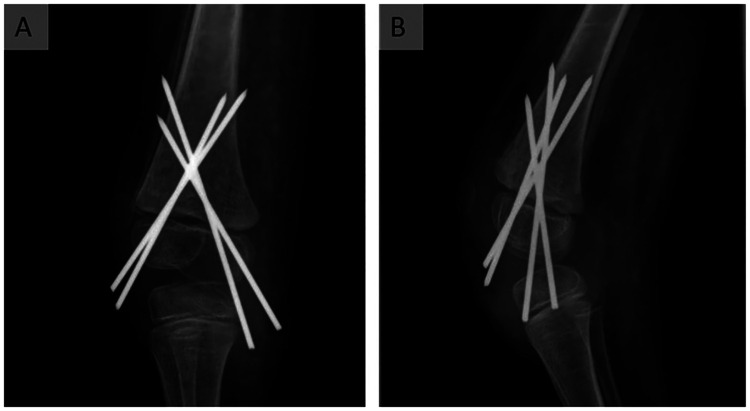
Follow-up radiograph of the right knee, 1 month after surgery. The radiograph shows a correct axial alignment of the epiphysis, 1 month after surgery of the Salter–Harris type 1 fracture in coronal (A) and sagittal (B) views. There are no signs of secondary dislocation or wandering of the K-wires. Mild osseous consolidation can be seen in (B).
